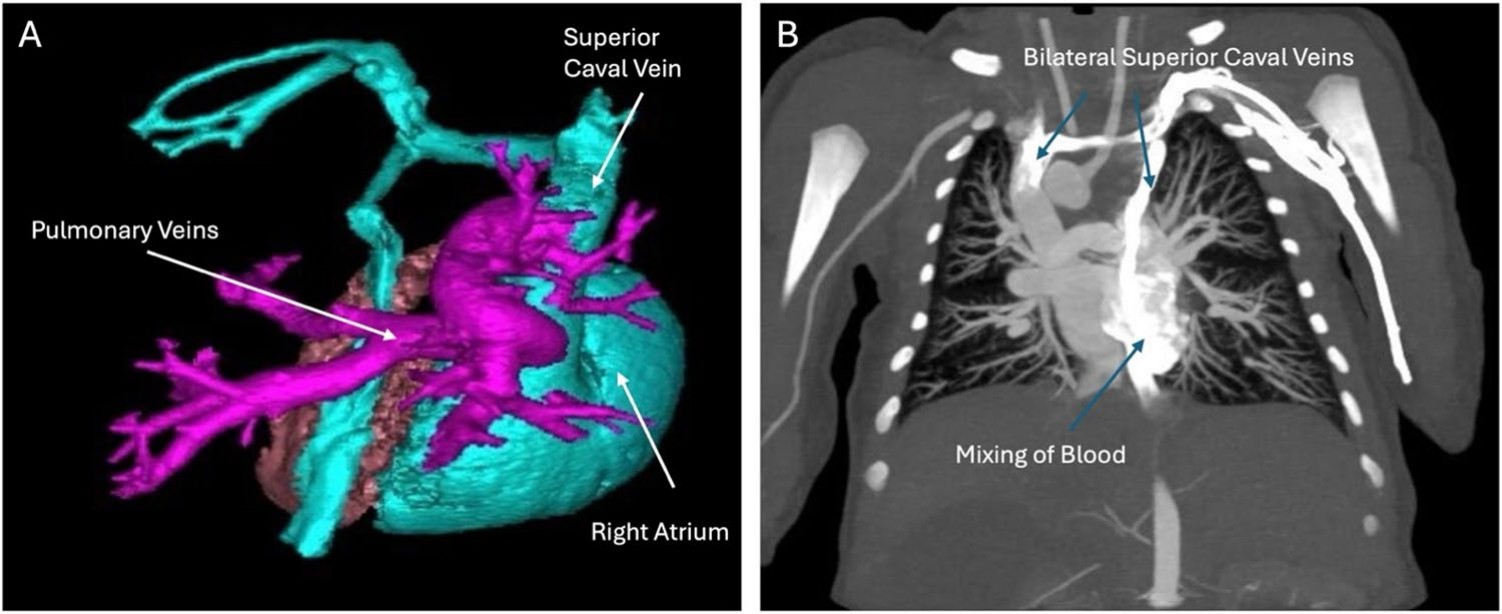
(A) Three-dimensional reconstruction showing total anomalous pulmonary venous return, with pulmonary veins emptying into the superior caval vein and right atrium. (B) Computed tomography angiography showing bilateral superior caval veins connecting to the atria, along with blood mixing due to lack of separation between the left and right ventricles
